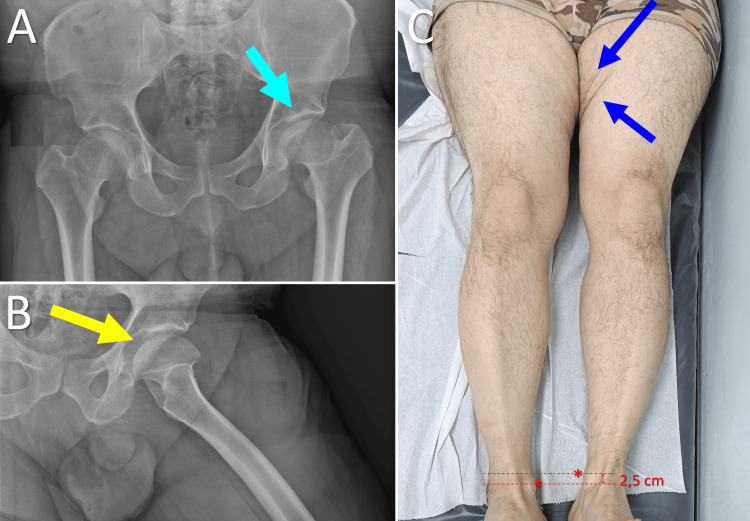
Asymmetric skin fold (ASF) formation in a 44-year-old patient with growth disturbance of the proximal femoral epiphysis and limb length inequality Α. Anteroposterior pelvic X-ray indicating bilateral growth disturbance of the proximal femur, more prominent on the left side (light blue arrow); B. Morphologic changes of the proximal femur are evident on the lateral x-ray view of the left hip (yellow arrow); C. Two ASFs (blue arrows) are formed on the 2.5 cm shorter left thigh (asterisks indicate the medial malleoli). Image credits: P. Samelis
